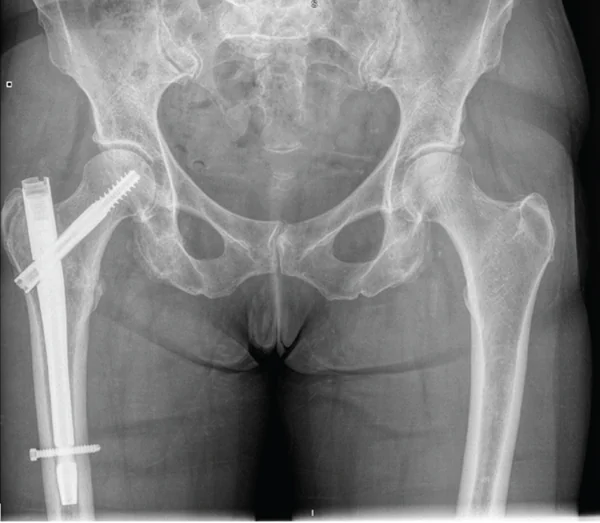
AP pelvic radiograph 6 weeks post‐surgery.
